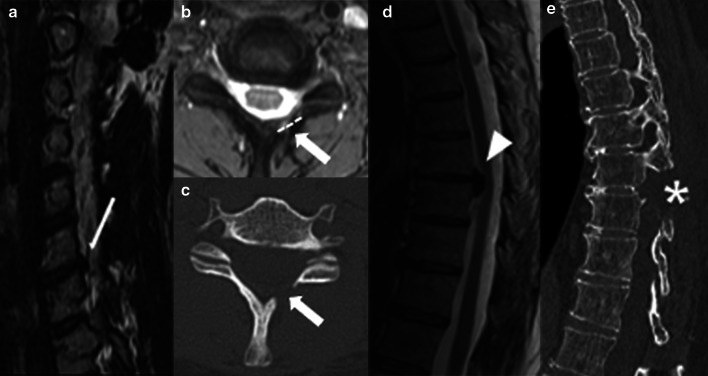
Discectomy and laminectomy. A disc herniation (thin arrow) at the C6-7 level is demonstrated on sagittal T2W preoperative MR image (a). Discectomy with laminectomy and flavectomy (arrows) were performed, as shown on axial GRE MRI (b) and CT image in bone window (c). Note that there is a larger resection area (dashed line) compared to laminotomy (Fig. 3B). A larger disc herniation (arrowhead) in another patient at the T8-9 level is shown on a sagittal T2W preoperative MR image (d). To gain a greater exposure to the vertebral canal, a discectomy using the laminectomy with medial facetectomy technique (asterisk) was performed, as demonstrated on the sagittal CT reformat (e). This procedure comprises in removal of the lamina and a partial resection of the facet joint
